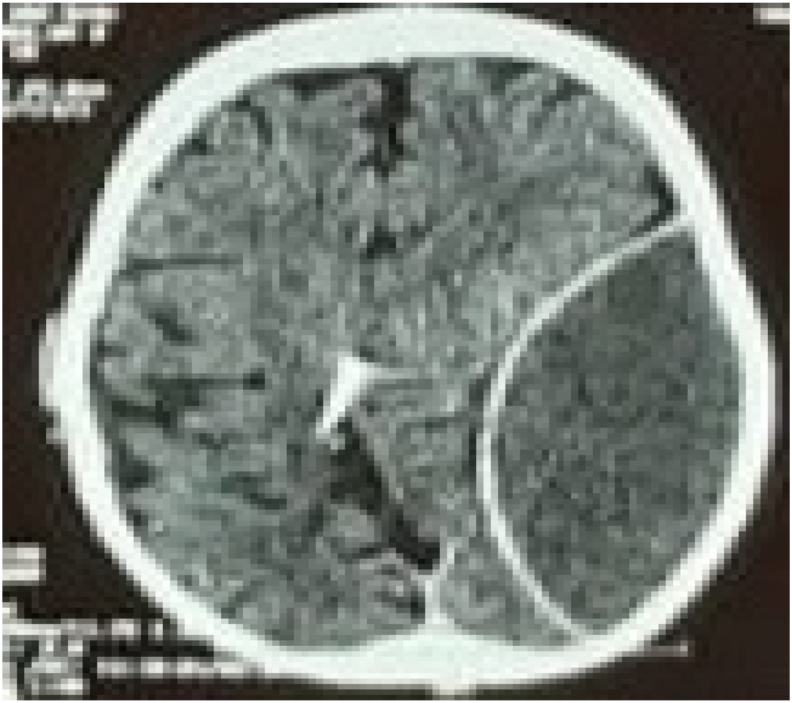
The CT scan of brain showing left extradural hematoma with mass effect. The ventricular catheter is seen within the right occipital horn.
